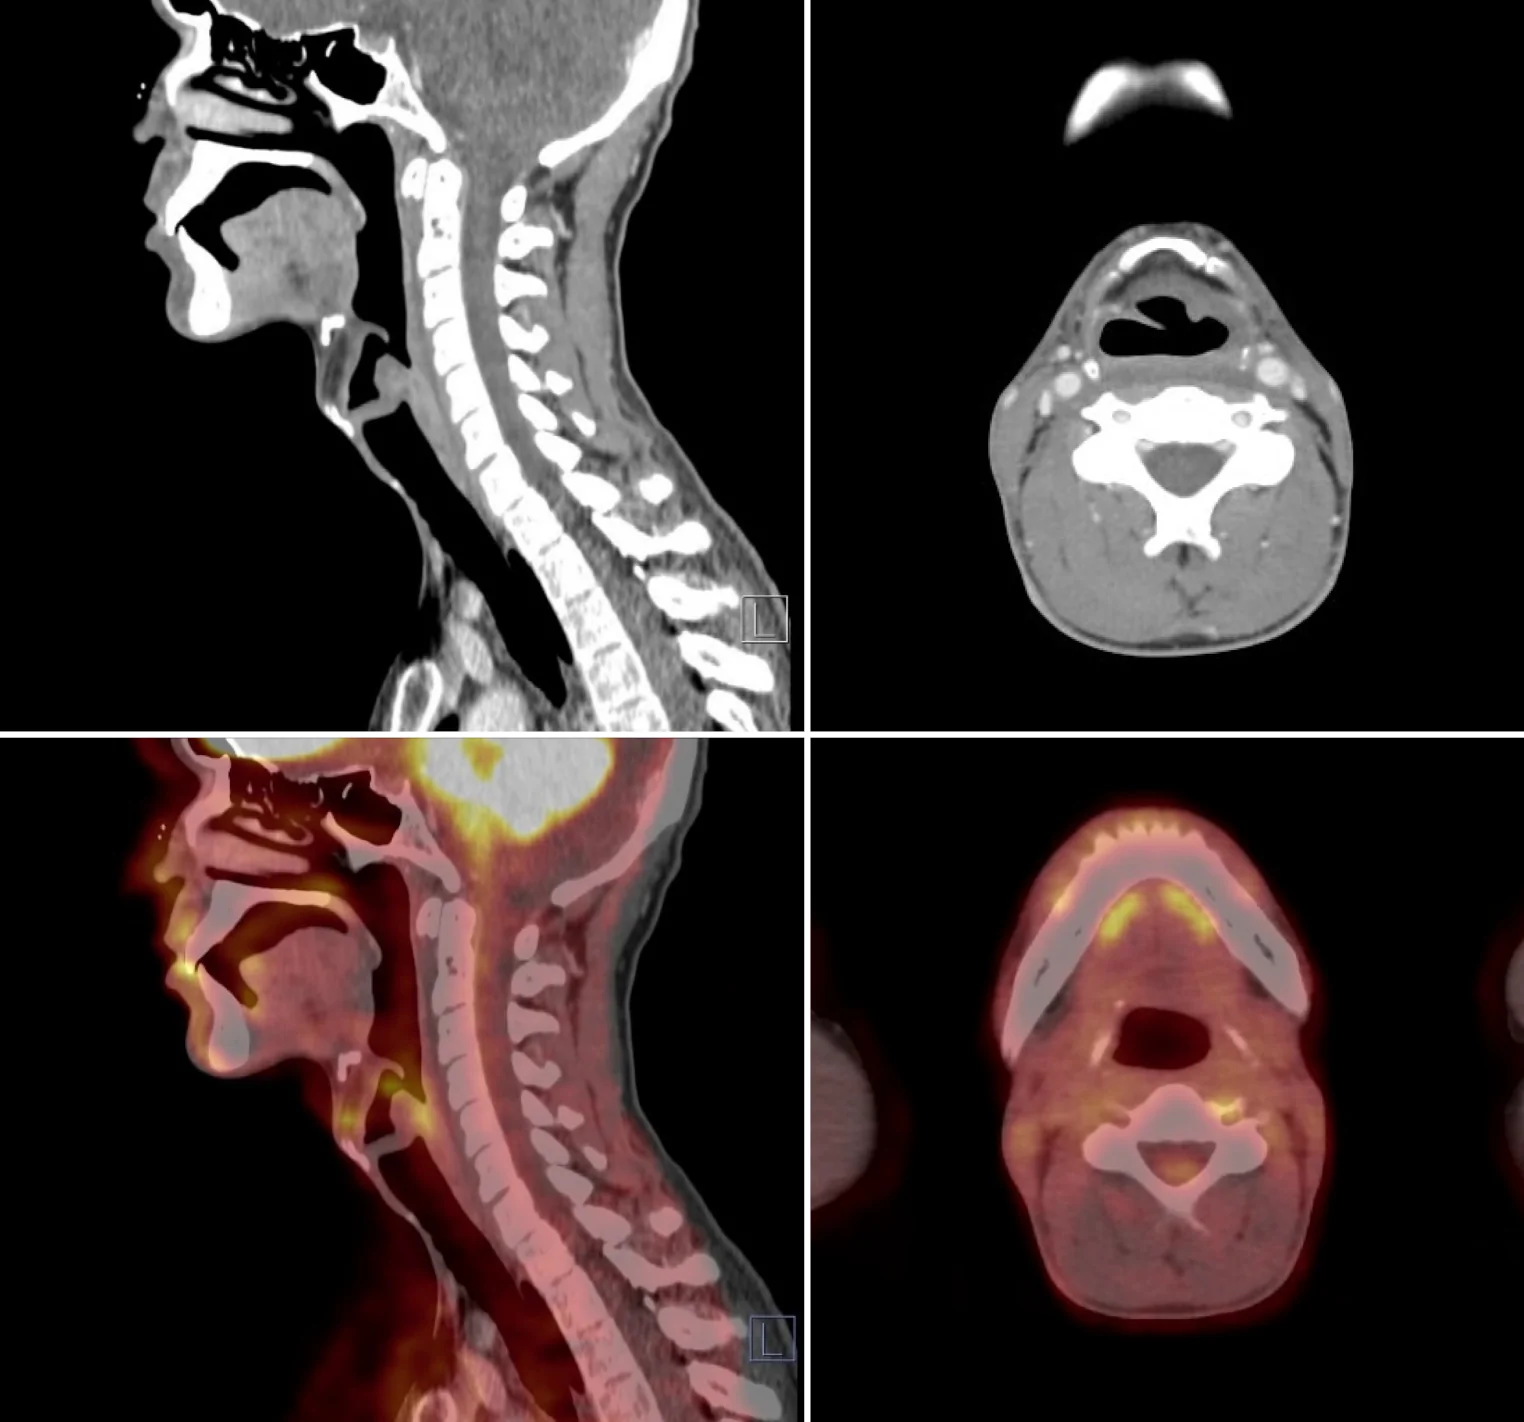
Five-year follow-up imaging after treatment. Contrast-enhanced CT demonstrates marked regression with no residual measurable disease. Corresponding fused FDG PET/CT shows complete metabolic response at the primary site and cervical lymph nodes
